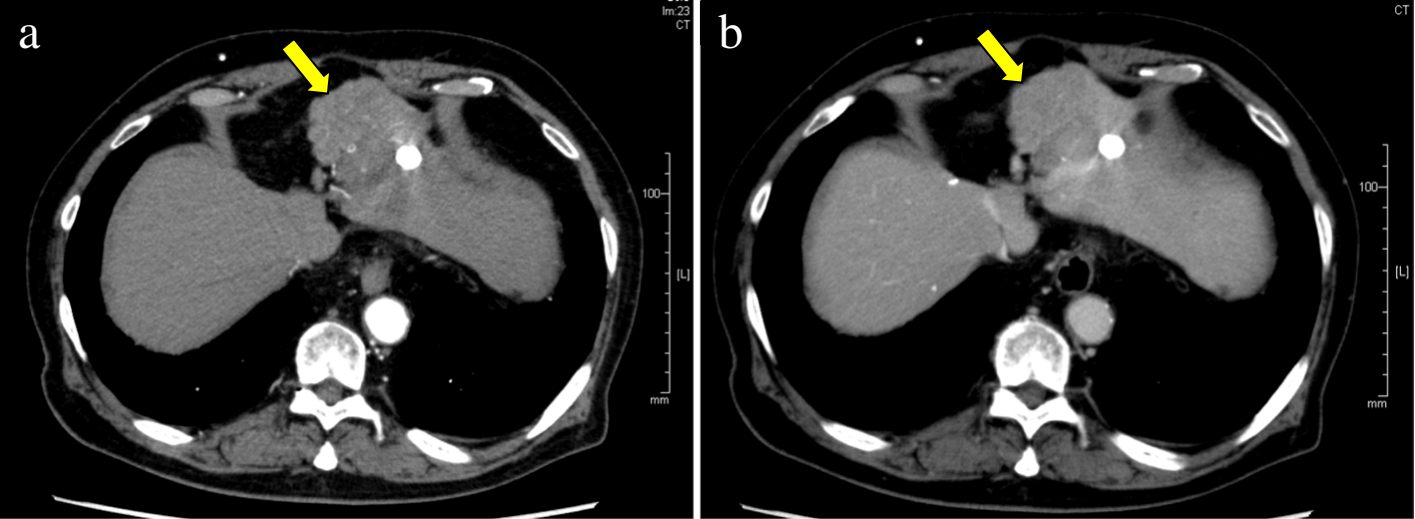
Enhanced computed tomography (CT) showed a recurrent lesion with radiofrequency ablation (RFA) and transcatheter arterial chemoembolization (TACE) scar inside (30 mm in diameter) at the surface of segment III. a Early arterial phase. b Delayed phase
